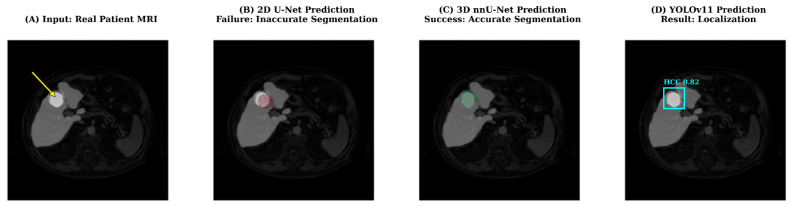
Qualitative failure analysis on a low-contrast HCC case. (A) Input MRI showing a subtle lesion (arrow). (B) 2D U-Net yields fragmented segmentation (red) due to the lack of volumetric context. (C) 3D nnU-Net successfully delineates the tumor (green) by leveraging inter-slice continuity. (D) YOLOv11 accurately localizes the lesion (cyan box), demonstrating robustness in detection tasks despite low contrast.
